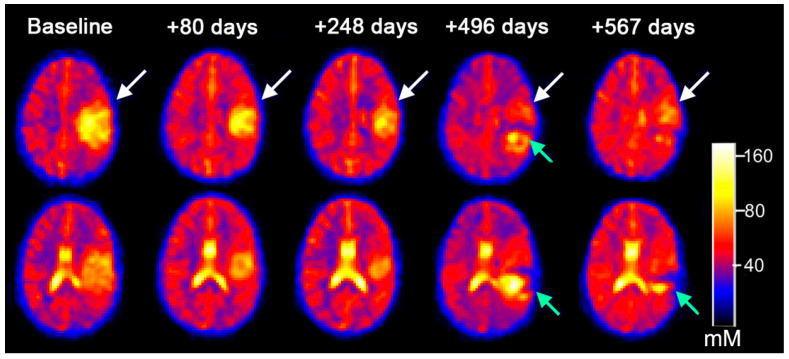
Serial total sodium MRI images at two different axial levels from a participant with supratentorial high-grade glioma treated with immunotherapy. This time series demonstrates a decrease in TSC relative to uninvolved tissue, preceding the eventual lesion size reduction in the tumor (white arrow) as noted by fluid-attenuated inversion recovery (FLAIR) imaging (Supplemental Figure S3). Note, a separate necrotic recurrent lesion (green arrow) also depicted in the sodium vBSC images (Supplemental Figure S5).
